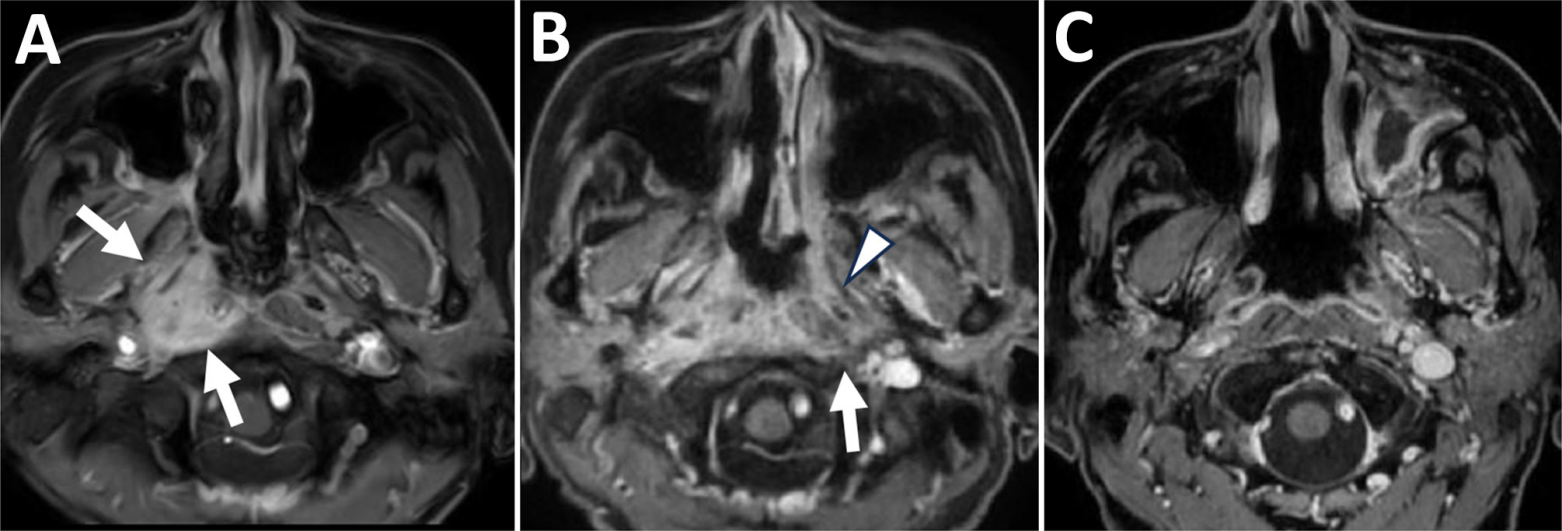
Chronological changes in lesions observed on contrast-enhanced fat-suppressed T1-weighted magnetic resonance imaging at the nasopharyngeal level in a patient in Japan with invasive chronic rhinosinusitis caused by *Bjerkandera adusta* fungi. A) Initial visit. Enhancement effects observed in the right nasopharynx, including the right torus tubarius and right prevertebral space (white arrows). B) Three months after the initial visit. Expansion of the enhancing lesion is seen, with enhancement extending to the left peritubal region (white arrowhead) and prevertebral space (white arrow). C) One year after the initial visit. The abnormal enhancement previously observed around both the torus tubarius and prevertebral space has regressed.
